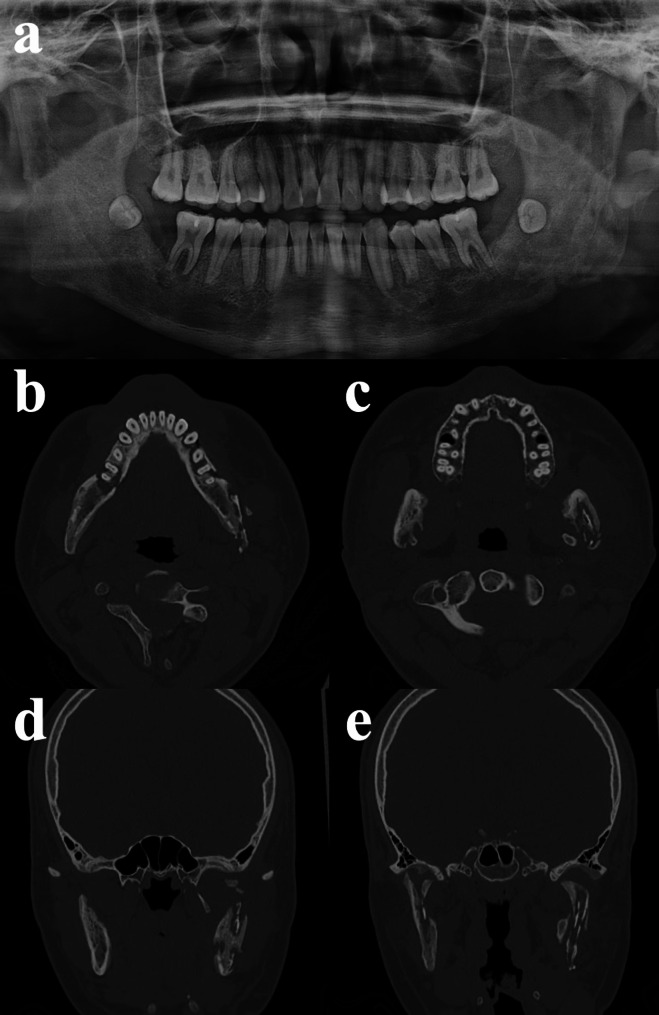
Two-month follow-up after discharge. a Panoramic radiograph. b, c Axial CT images. d, e Coronal CT images
